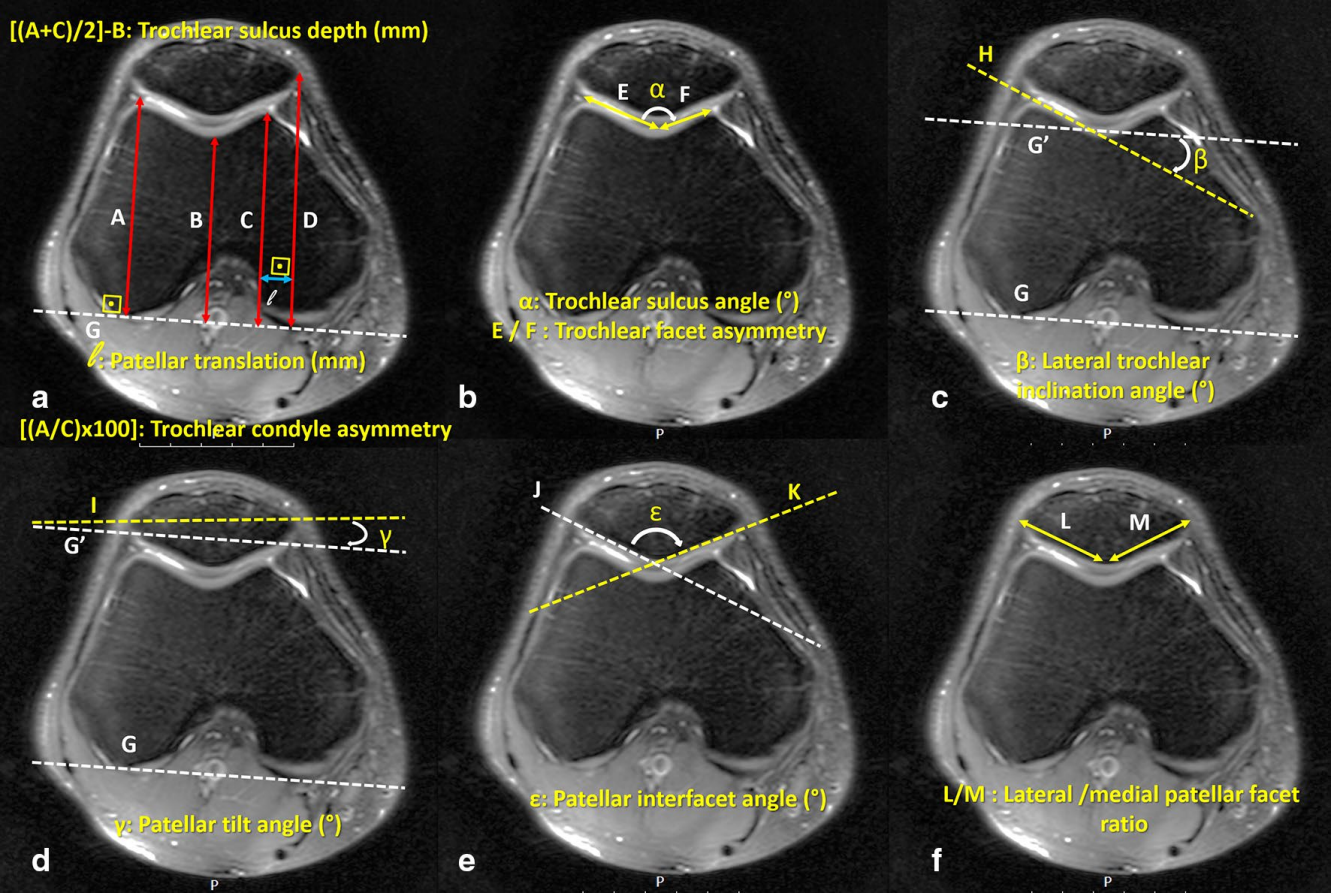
a Measurement of trochlear sulcus depth, patellar translation, and trochlear condyle asymmetry. First, posterior condylar axis was drawn (line G). Perpendicular lines from the highest points of the medial (line A) and lateral (line C) facets, and the deepest point (line B) of the trochlear groove were drawn. Finally, the perpendicular distance from the posterior condylar axis to the medial corner of the patella (line D) was drawn. Trochlear sulcus depth was calculated as [(*A* + *C*)/2] − *B* and recorded in millimeters. Patellar translation was measured as the perpendicular distance between line C and line D and recorded in millimeters. Trochlear condyle asymmetry was calculated as [(*A*/*C*) × 100%] and recorded as a percentage. b Measurement of trochlear sulcus angle and trochlear facet asymmetry. Two parallel lines, one to the lateral facet of the trochlea (line E) and the second one to the medial facet of the trochlea (line F), were drawn. The angle (*α*) between these lines was measured as the trochlear sulcus angle. The ratio of the distance line E to line F was calculated as the trochlear facet asymmetry. c Measurement of lateral trochlear inclination angle (*β*) between the lateral trochlear slope (line H) and posterior condylar axis (line G). d Patellar tilt was defined as the angle (*γ*) formed between the transverse axis of patella (line I) and posterior condylar condyle line (line G). e Patellar interfacet angle was defined as the angle between the lateral patellar facet (line K) and medial patellar facet (line J). f The ratio of the lateral (line M) to the medial (line L) patellar facets was calculated. All measurement were performed on axial fat-saturated, proton-density-weighted MRI
